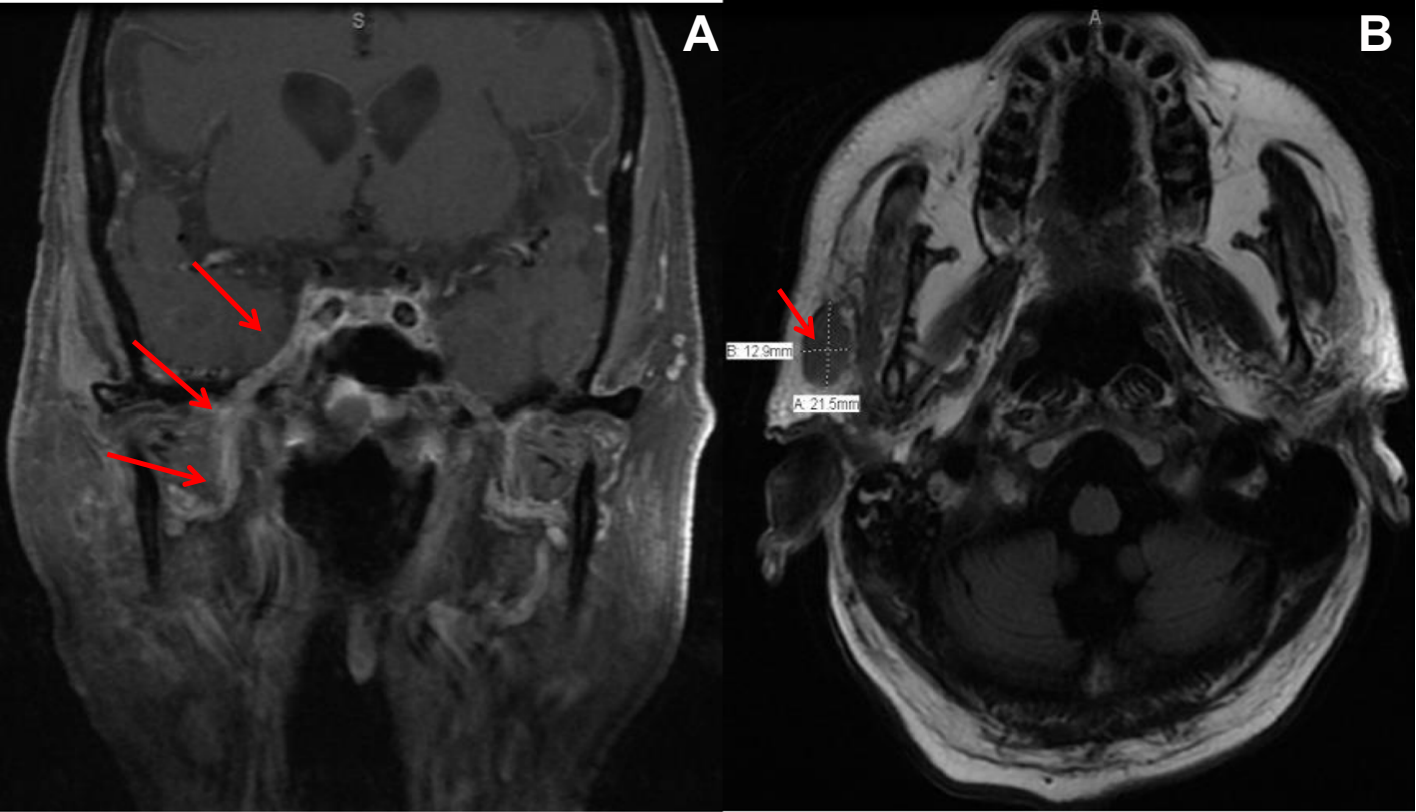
MRI Brain and orbit with and without contrast. A: asymmetric enhancement in the right Meckels cave along the V3 division of the trigeminal nerve suggestive of perineural spread of tumor. B: overlying the right parotid gland, there is a new 22 × 13 mm heterogeneously enhancing lymph node.
